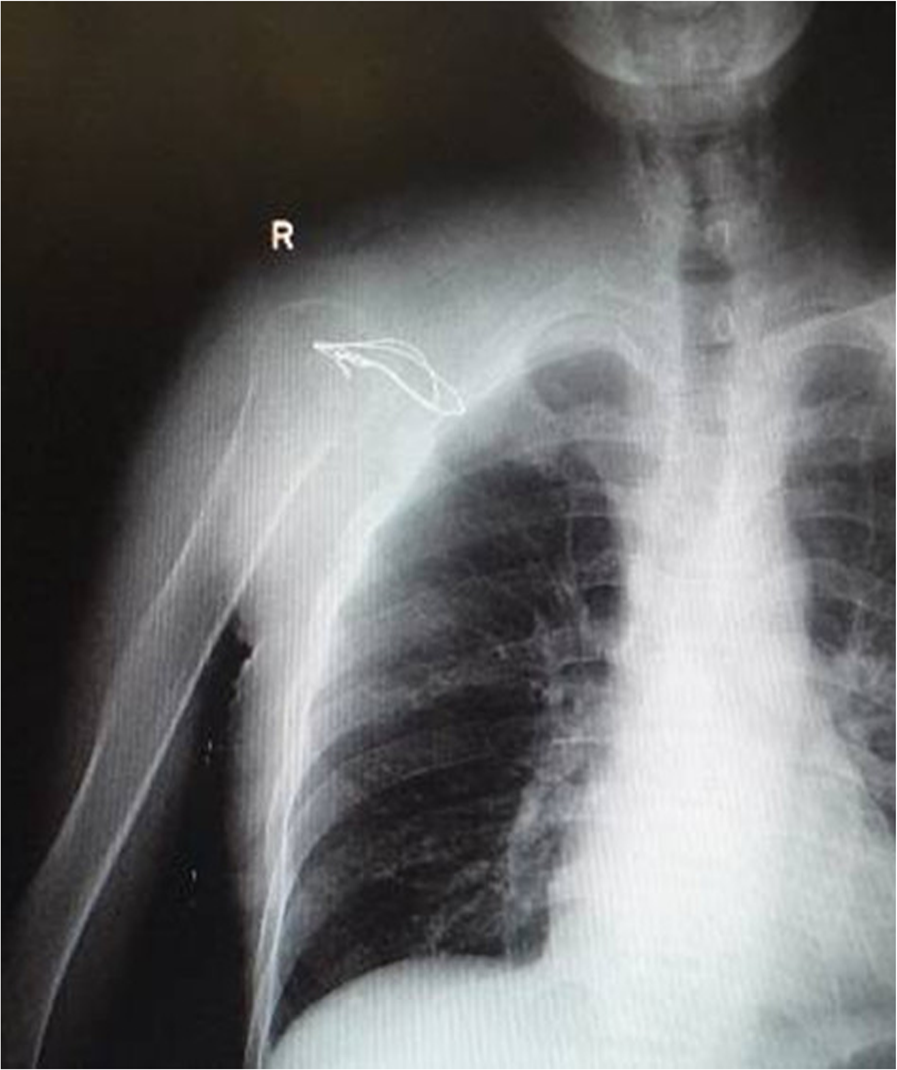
The postoperative radiograph of the right shoulder.
